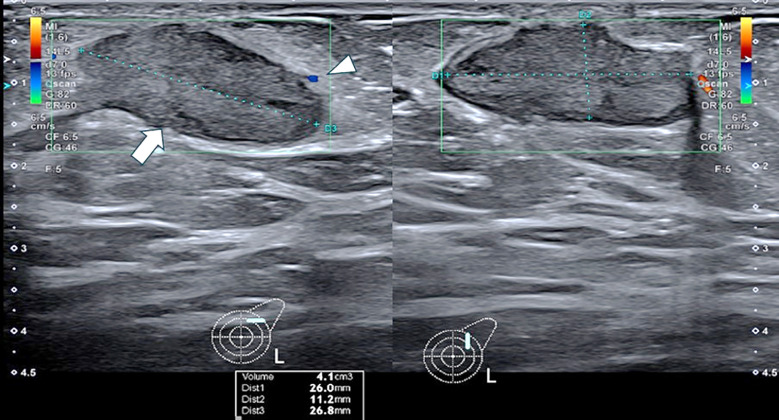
Ultrasound image of an elderly female patient with recurrent non-lactational breast abscess showing a dilated duct with homogenous, diffuse intraluminal echoes without vascularity at the 1 o’clock position (arrow). Surrounding increased echogenicity of parenchyma (arrowhead) is reactionary.
